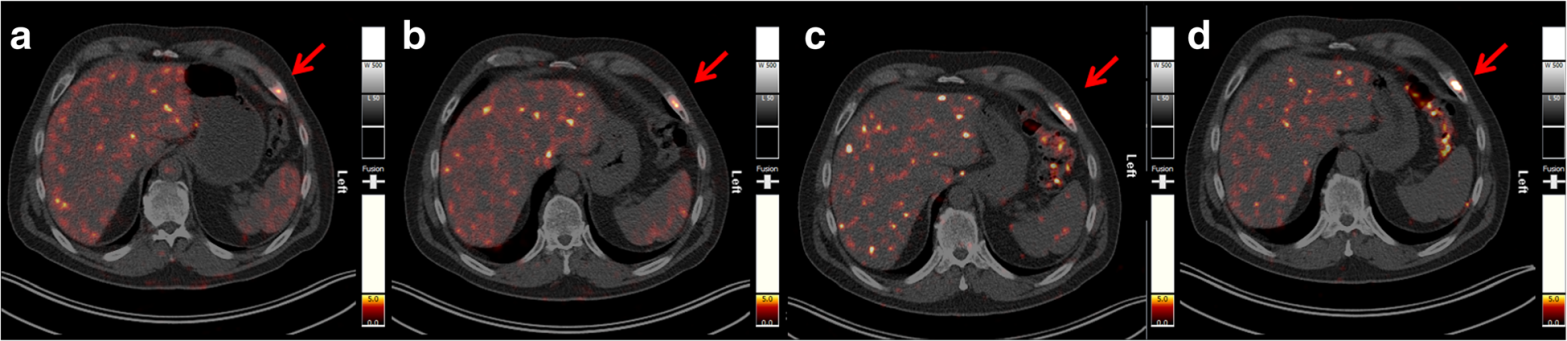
PET/CT scans (transversal slices through the upper abdomen at the level of the liver and spleen) obtained at a 50 min, b 2 h, c 18.5 h, and d 25 h, respectively after injection of 140 MBq ^152^Tb-PSMA-617, clearly demonstrating a PSMA-avid bone metastasis in the ventrolateral part of the left 7th rib (red arrow) with maximum uptake at 18.5 and 25 h p.i.
